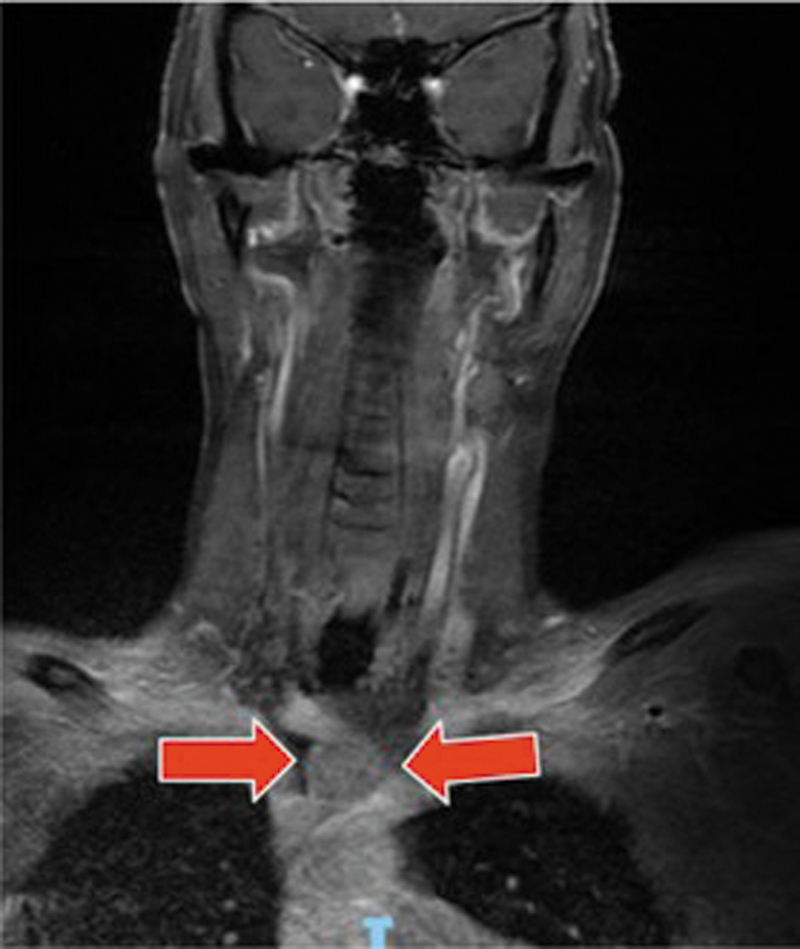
Coronal T1-weighted contrast-enhanced MRI demonstrating a well-defined nodular lesion in the anterior mediastinum (red arrows), consistent with thymoma.
